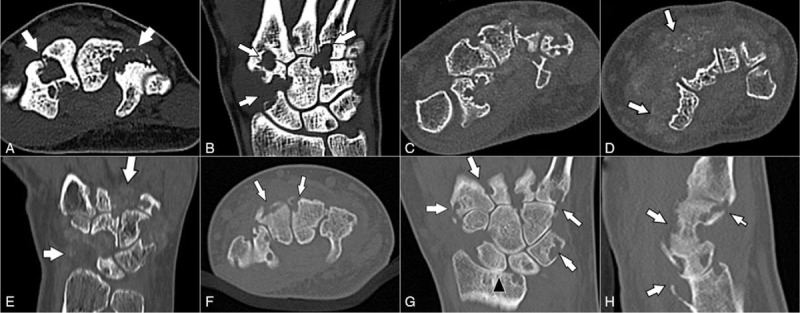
Computed tomography images of the 3 patients. In Case 1, the axial (A) and coronal (B) images reveal multiple erosions of the carpal bones and metacarpal bases (arrows). The axial (C and D) and coronal (E) images of Case 2 showed multiple erosions of the carpal bones and metacarpal bases; surrounding, ill-defined, lobulated, soft tissue mass-like lesions show stippled calcification (arrows). The axial (F), coronal (G), and sagittal (H) images of Case 3 demonstrate multiple erosions of the radius, carpal bones, and metacarpal bases (arrows), with narrowing of the radiocarpal joint space (arrowhead).
